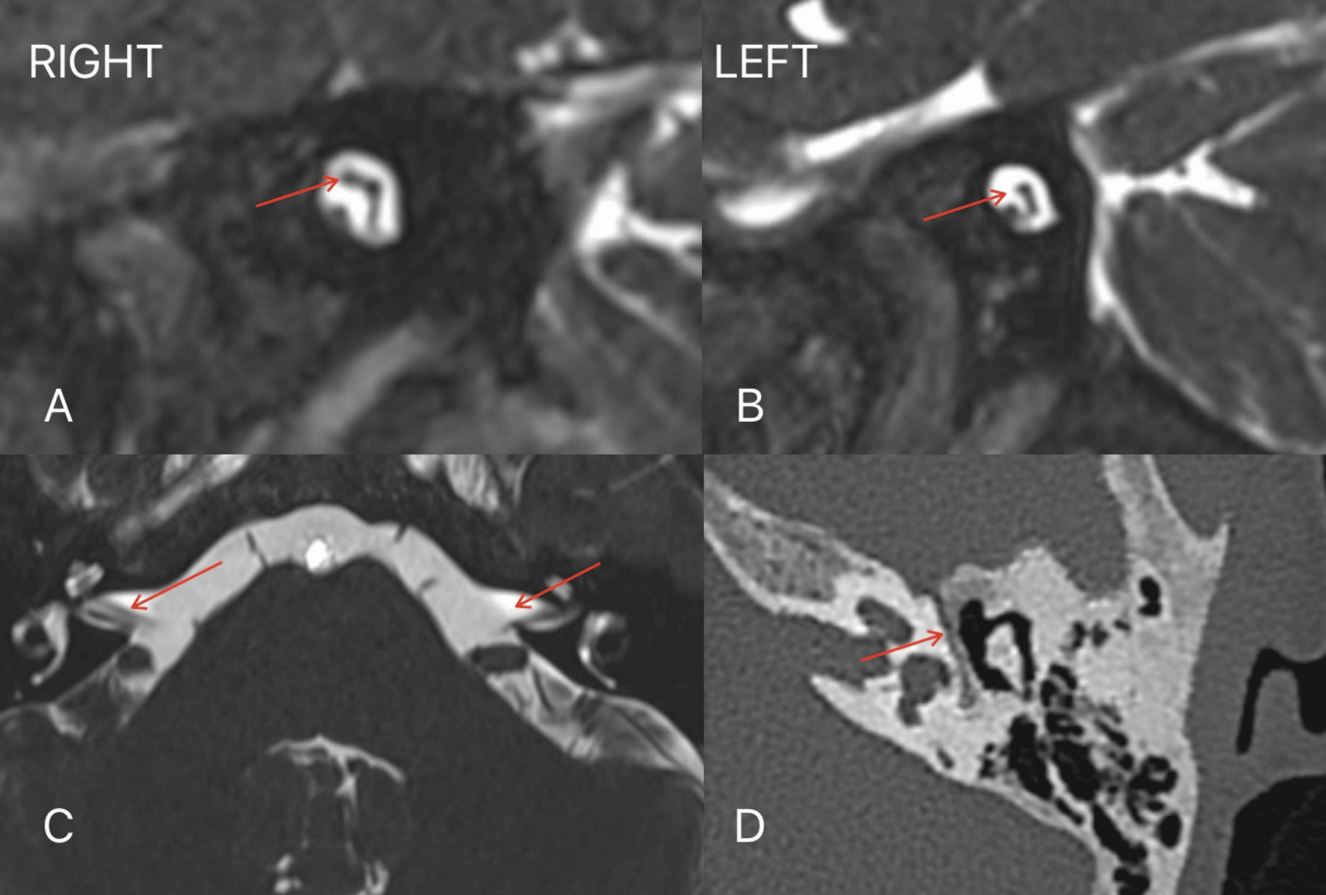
(A) Oblique sagittal 3D CISS MRI sequence along the right internal auditory canal (IAC) - normal right facial nerve in the anterosuperior quadrant of the right IAC (arrow). (B) Oblique sagittal 3D CISS MRI sequence along the left IAC showing an empty anterosuperior quadrant of the left IAC (arrow) with a comma-shaped vestibular nerve complex. (C) Posterior fossa axial 3D CISS MRI sequence showing normal cisternal segment of the right facial nerve (arrow) with absence on the left side. (D) High-resolution CT showing a normally developed bony left facial canal (arrow). CISS: Constructive interference in steady state.
